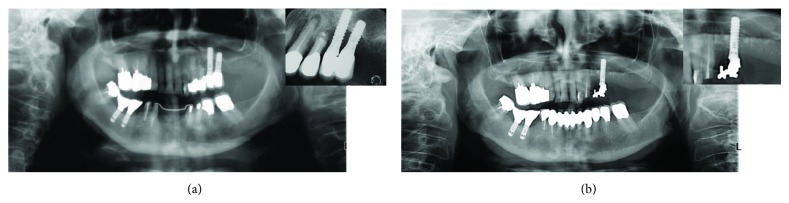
Comparison between periapical and panoramic radiographs before and after 12 months from surgery.
